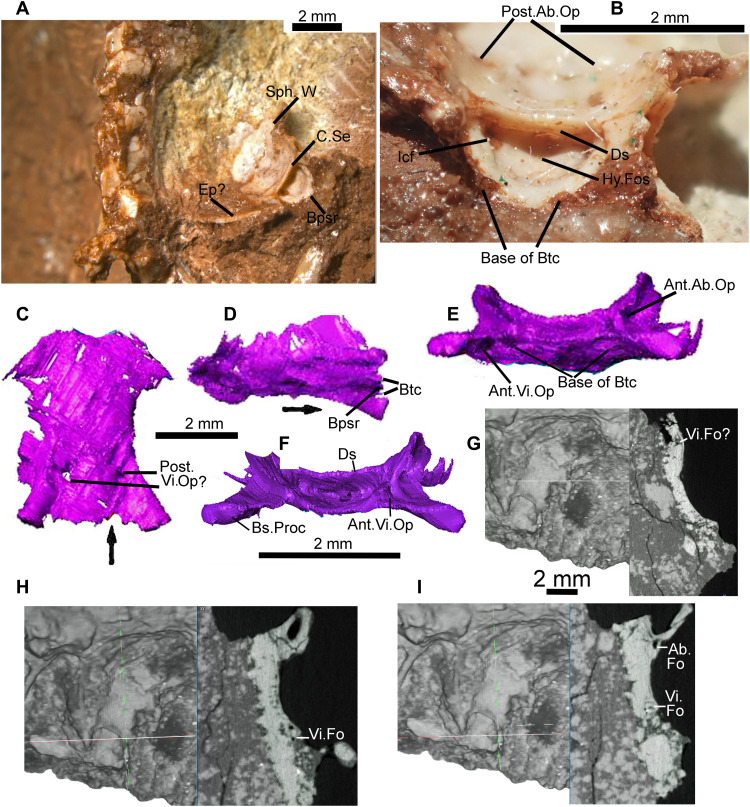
Images of the holotype of *C. microlanius* showing sphenoid and indicating squamate features. (A) View of holotype highlighting the sphenoid with a prominent dorsum sella (showing a deep fossa) and high crista sellaris. (B) Close-up anterior (and slightly dorsal) view of the sphenoid showing posterior opening of the abducens canal and internal opening of the carotid canal. (C to F) CT scan of sphenoid in (C) ventral, (D) right latero-ventral, (E) antero-ventral, and (F) anterior views. (G to I) CT cross sections on right of each image, with thin white line showing position on left-hand side, which is a dorsal view. (G) is about midway antero-posteriorly with the slice cutting across the lateral “wing.” (H) and (I) are at the anterior of the sphenoid; (I) is the most anterior. Squamate features in (C) to (I) show position of Vidian and Abducens canal foramina. All scale bars, 2 mm [bar for (C) and (D) between images; (E) and (F) bar under (F) and bottom bar is for (G) to (I)]. Ab, abducens; Bpsr, parasphenoid rostrum; Btc, trabeculae cranii; Ds, dorsum sella; Ep, epipterygoid; Hy, hypophysial; Icf, internal carotid foramen; Se, sellaris; Vi, vidian; W, wing.
